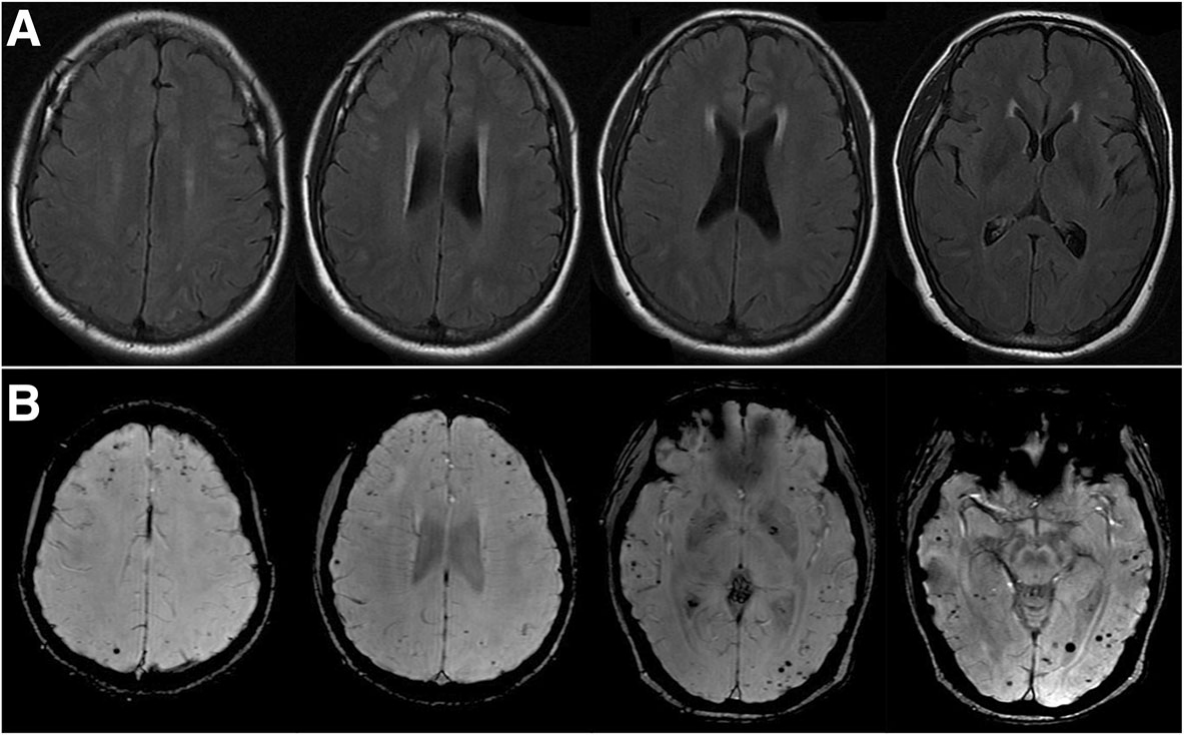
MRI findings after the first corticosteroid therapy. a FLAIR images showing lesions were almost completely disappeared; b SWI images showing multiple cerebral microbleeds remained unchanged
